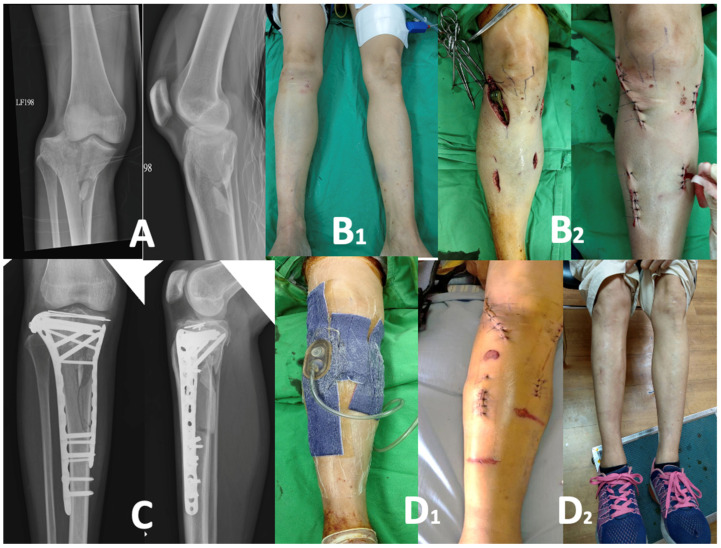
One-stage ORIF with plate tibia plateau fracture with dislocation. (A) A 38-year-old female sustained Schatzker type IV tibial plateau fracture with dislocation due to a scooter accident. Plain films of the injury are shown. (B1) At presentation, the soft tissue status is relative stable even though swelling has been found. (B2) ORIF is performed as a one-stage surgery. The wound used is shown above. (C) Plain films of ORIF are shown, with good reduction and fixation. (D1) Application of closed-incision negative-pressure therapy for the operative wound immediately postoperatively and the removal of the wound therapy device 5 days after its application. (D2) Right knee wound has healed nicely 6 months after ORIF.
